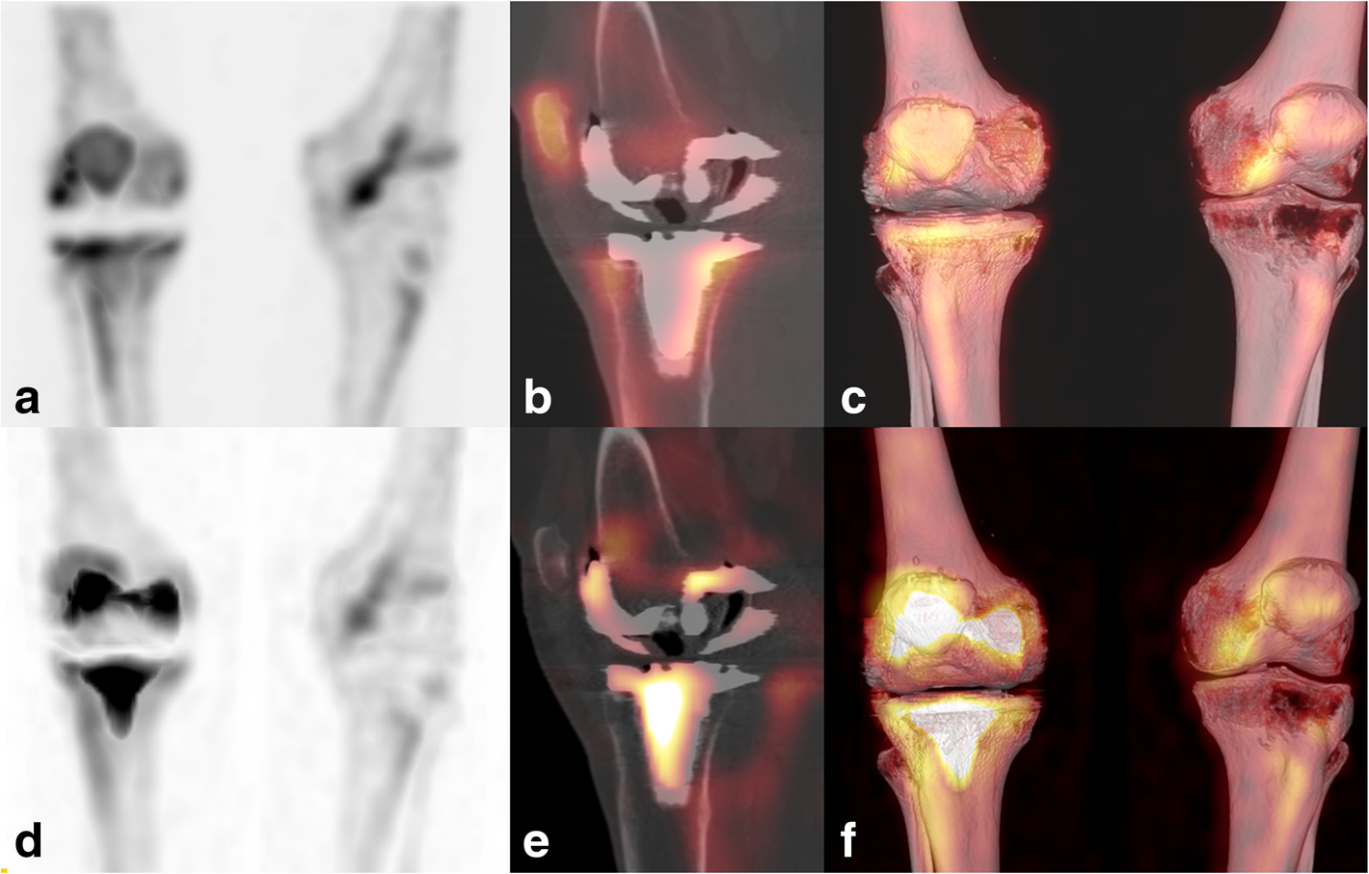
xSPECT Bone “shining metal artefact” in the knee. SPECT/CT images of a 77-year-old female patient 17 years after insertion of a knee arthroplasty. MIP image with xSPECT Bone reconstruction (d), fused sagittal SPECT/CT (e) and fused 3D reconstruction (f) show high “pseudouptake” of the tibial and femoral arthroplasty components. Corresponding images (a–c) with conventional Flash3D reconstruction show no such abnormal radiotracer uptake in and around the metal implants but only true increased uptake in the adjacent bone and patella. Conservative treatment with physiotherapy was initiated after the SPECT/CT
